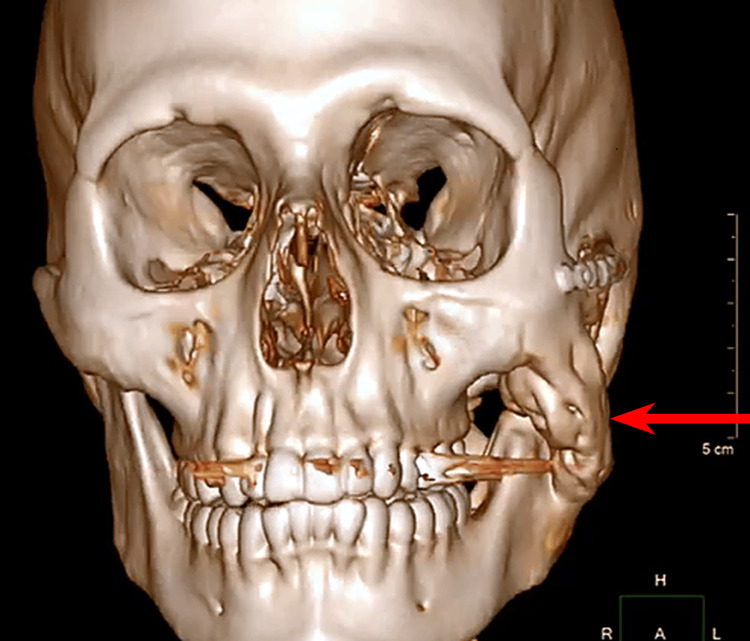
3D CT reconstruction (frontal view) showing heterotopic ossification in the left masseter muscle (red arrow).
